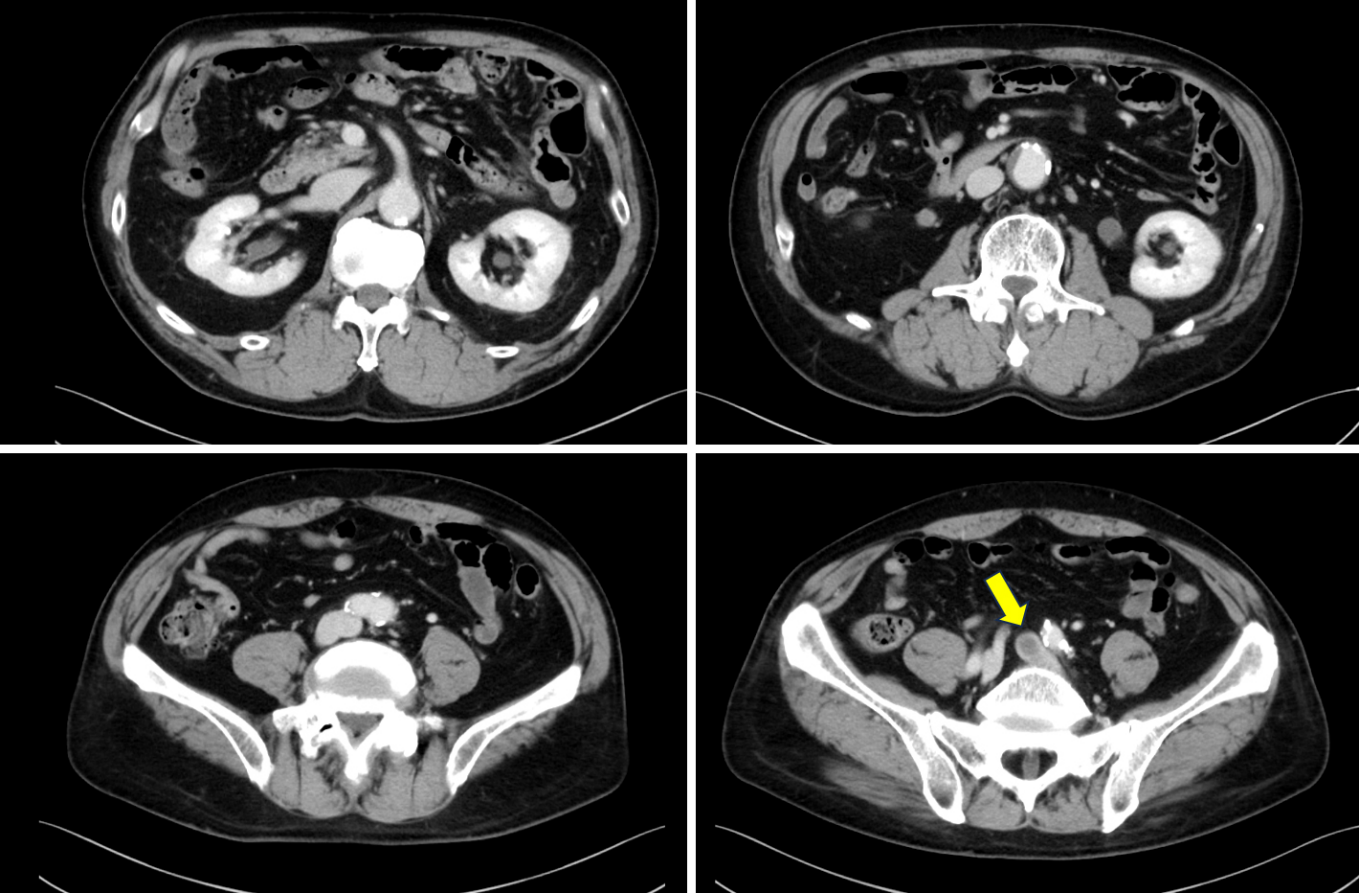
Past contrast-enhanced computed tomography showing a non-calcified thrombus in the left common iliac vein.
